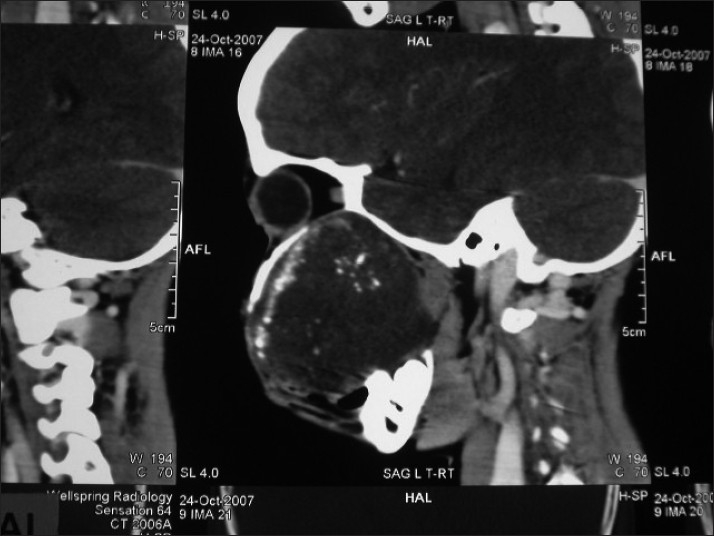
Computed tomographic image showing a single large expansile radiolucent lesion with multiple radioopaque foci
